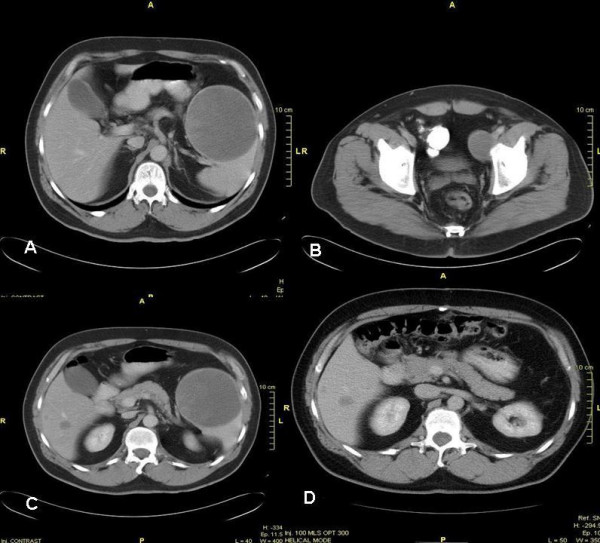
Computed tomography (CT) of the abdomen and pelvis. CT scanning immediately before initial pelvic tumor debulking at 46 months after presentation shows A). Large splenic metastasis (100.3 mm), B). Metastasis to the left iliac vein lymph nodes (41.3 mm), and C). A slightly lower view of the splenic metastasis showing the 2 liver lesions. D). CT of the abdomen, post-splenectomy and pelvic tumor debulking, and pre-liver resection (2 liver metastases 20 mm and 15 mm in segments V and VI) at 51 months.
